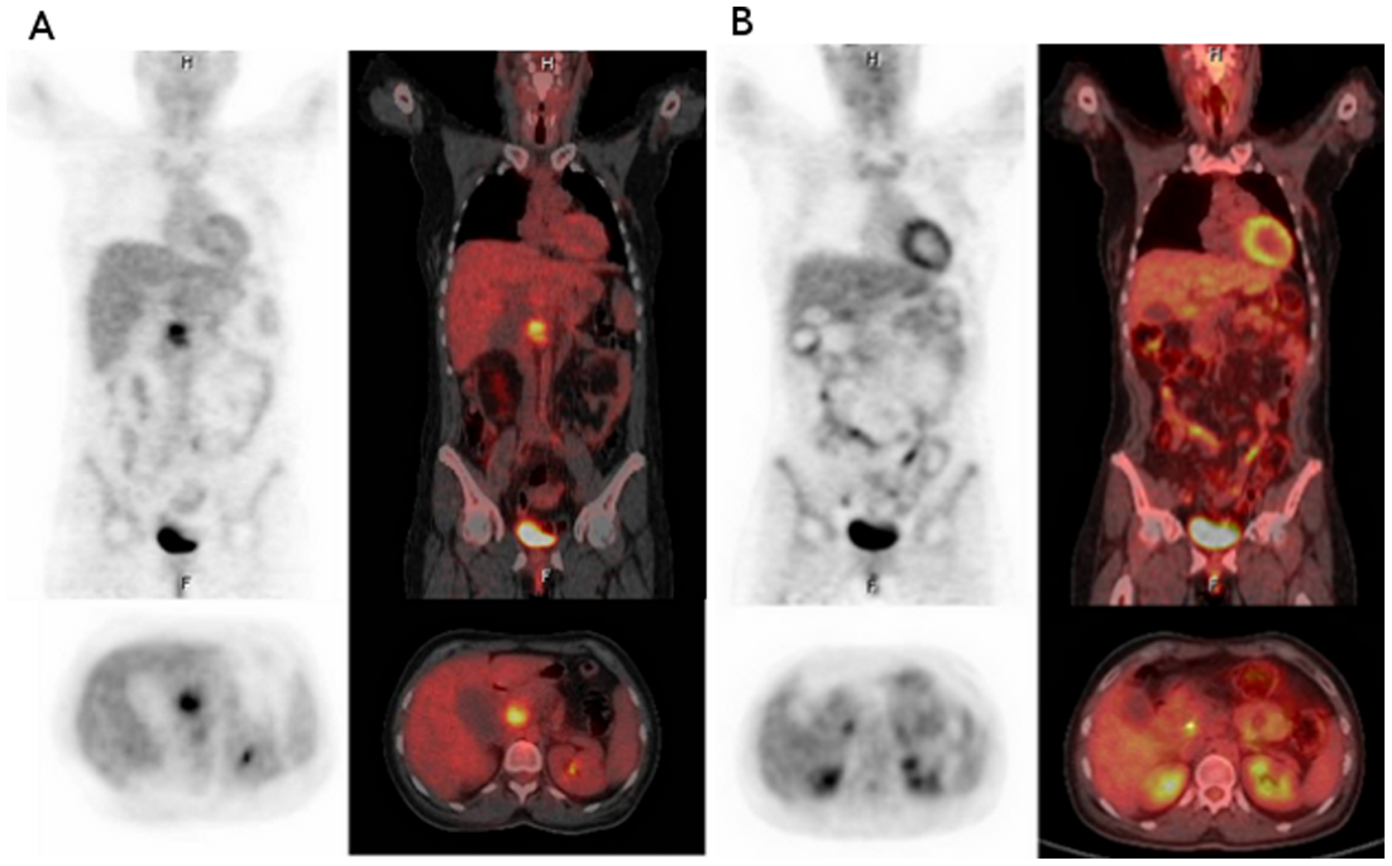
Positron emission tomography-Computed tomography images show pathological uptake (SUVmax = 4.59) in a 20×38 mm mass in the pancreatic body at baseline (A). There is no evidence of metabolic activity in the follow-up study (B), considered a complete response by EORTC criteria (PERCIST criteria).
